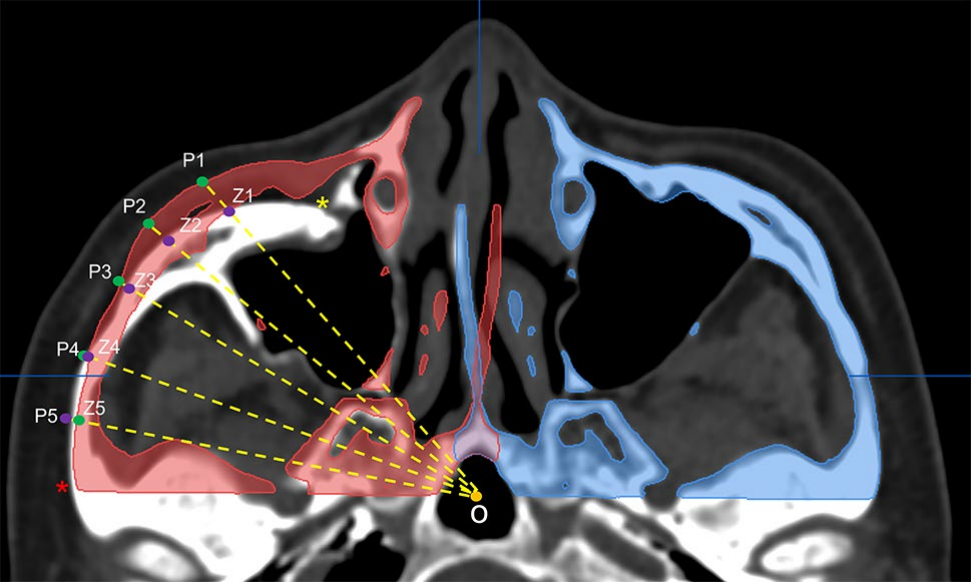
Measurements of zygoma conformity according to presurgical planning. The infraorbital foramen (yellow asterisk) and base of zygomatic arch (red asterisk) were identified on CT. The five points (Z1, Z2, Z3, Z4, and Z5) (violet points) on the zygomatic surface were defined and evenly distributed based on its angle of degree. The O point (yellow point) is defined as the intersection of the vertical midline and the horizontal line that passed through the bilateral zygoma arch base. The Z1O line (yellow dotted line) that intersected at the surface of the zygoma object during presurgical planning is defined as P1 (green point). The other points P2 to P5 (green points) are defined in the same manner. The preoperative and postoperative distances to virtual planning images between Z1 and P1, Z2 and P2, Z3 and P3, Z4 and P4, and Z5 and P5 were measured.
